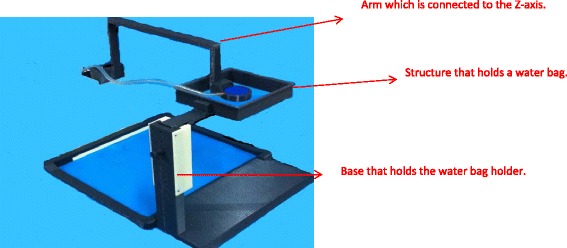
The developed coupling that can be used for a top-to-bottom access of ultrasound to targets
